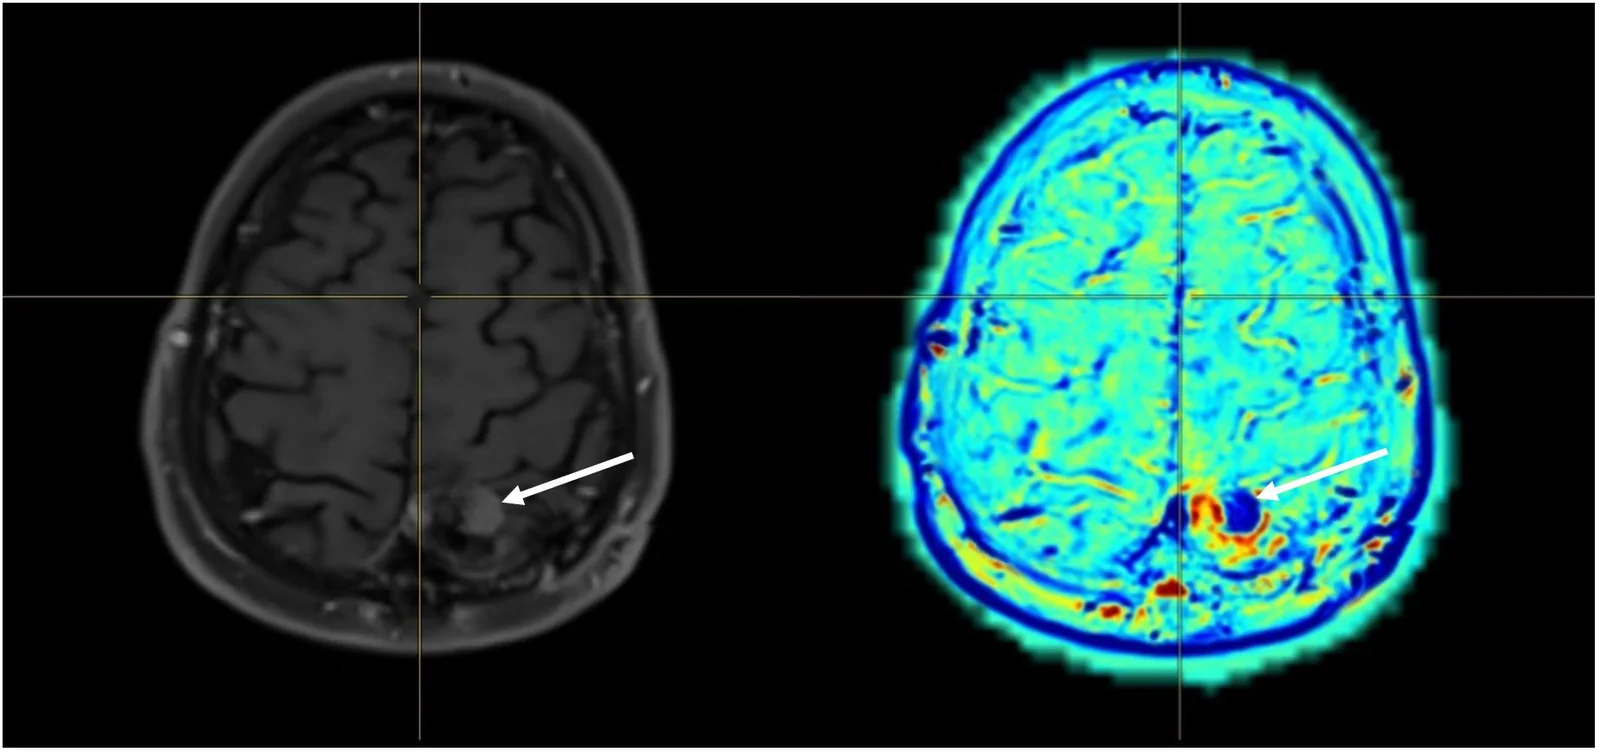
(A) Follow-up contrast-enhanced T1-weighted MRI at 7 months after surgery demonstrating local recurrence. (B) Corresponding to follow-up TRAMs/CCA confirming contrast clearance within the recurrent lesion.
